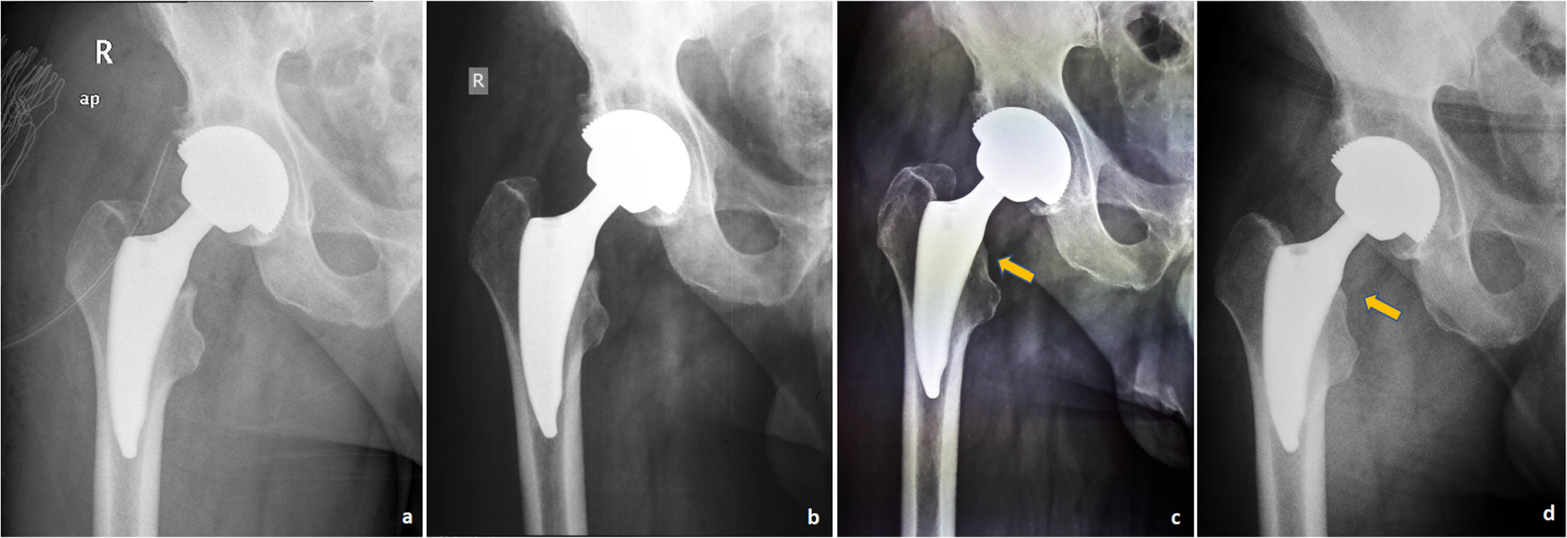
Anteroposterior pelvis radiographs of a 61-year-old man who underwent a right total hip arthroplasty with the Minima S stem. a First day, b 6 months, c 1 year, and d 2 years postoperatively. Grade 1 stress shielding effect was noted postoperatively at 1 and 2 years after implantation
